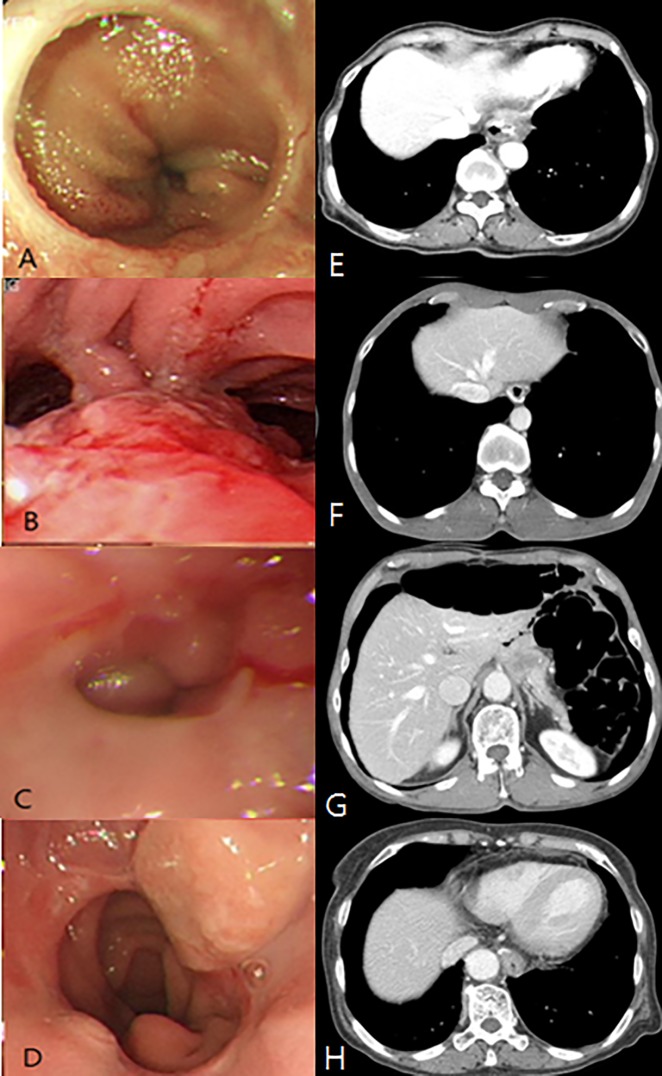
Endoscopic and contrast abdominal CT findings of peri-anastomotic tumor recurrence in patients diagnosed with AGC (each horizontal row of photos are from the same patient). A. Obstruction below the anastomosis site due to external compression B. Hyperemic and slightly elevated erosion, below the anastomosis site (missed by follow-up contrast abdominal CT scan) C. Stricture of the anastomosis site D. Subepithelial-like mass lesion, above the anastomosis site E. Prominent soft tissue density around the peri-anastomosis site F. No definite abnormal findings around the anastomosis site (missed by follow-up contrast abdominal CT scan) G. Low attenuation wall thickening at the anastomosis site H. Mild wall thickening just above the anastomosis site.
